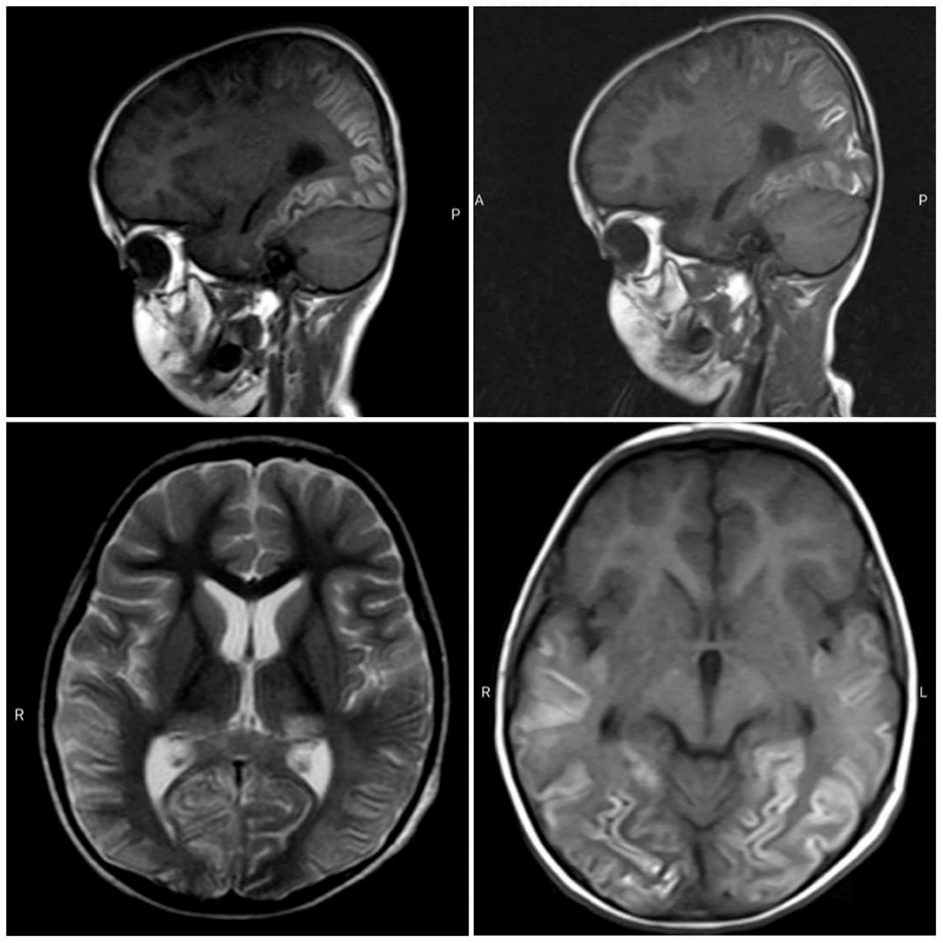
MRI obtained from brain. Images of brain MRI proposed mostly symmetric, cortical, and juxta-cortical high T1 and T2 signal abnormality, in bilateral parieto-occipital lobes.
